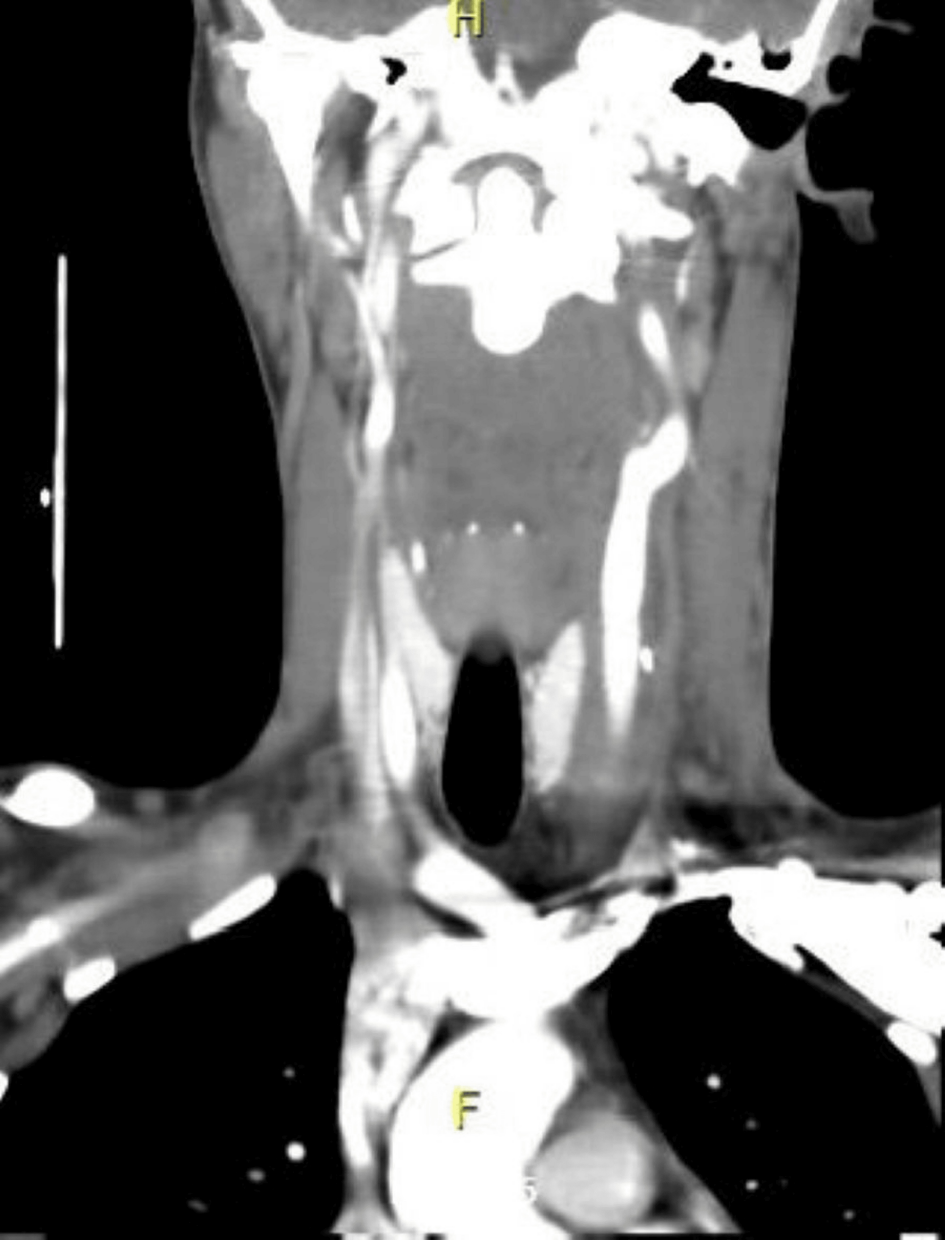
Postoperative CT (coronal plane). Postoperative changes with no obvious contrast extravasation, dissection, or wall irregularities were observed.
